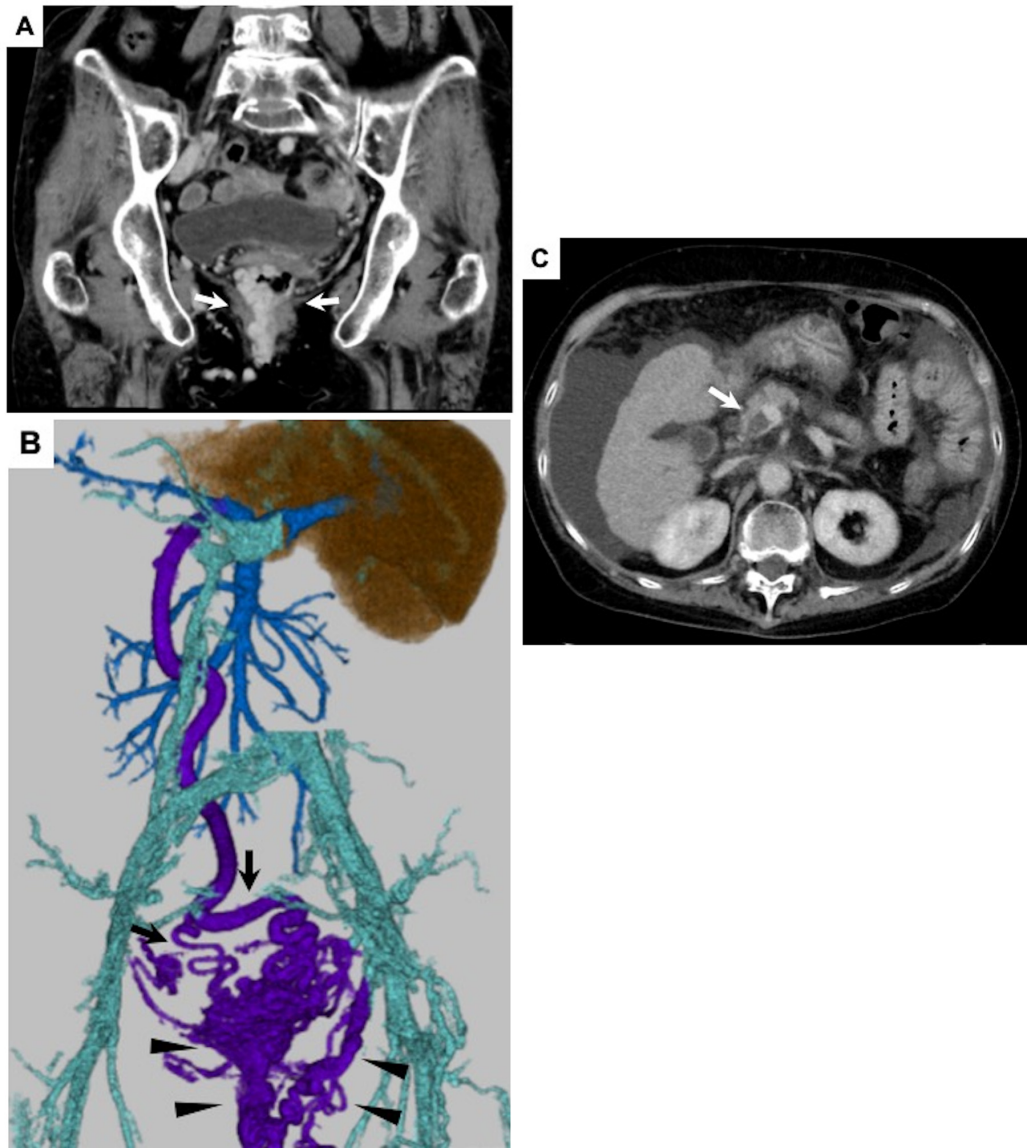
(A) Contrast-enhanced computed tomography (CECT) in coronal view revealed huge anorectal variceal formation (arrow). (B) Volume rendering of CECT (rear view) showed portosystemic collateral pathway where portal venous blood flowed from the bilateral superior rectal veins (arrow) to the anorectal varices with multiple draining veins (arrowhead). (C) CECT in axial view depicted severe liver cirrhosis with portal vein thrombosis (arrow) and ascites.
